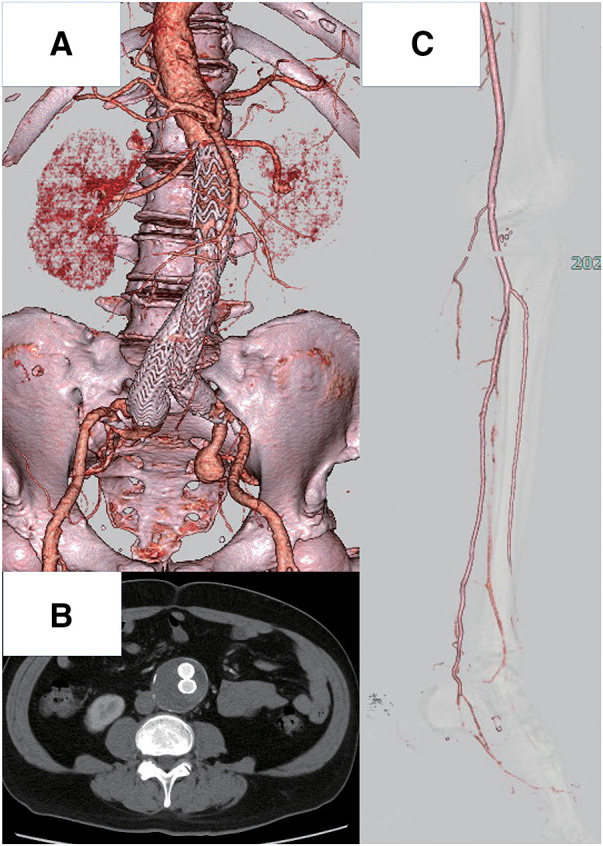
Postoperative CT scans. (A) CT angiography after EVAR. (B) An axial image at the same level as that in Fig. 1B. (C) CT angiography of the left limb. Arteries below the knee can be detected. CT: computed tomography; EVAR: endovascular aneurysm repair
